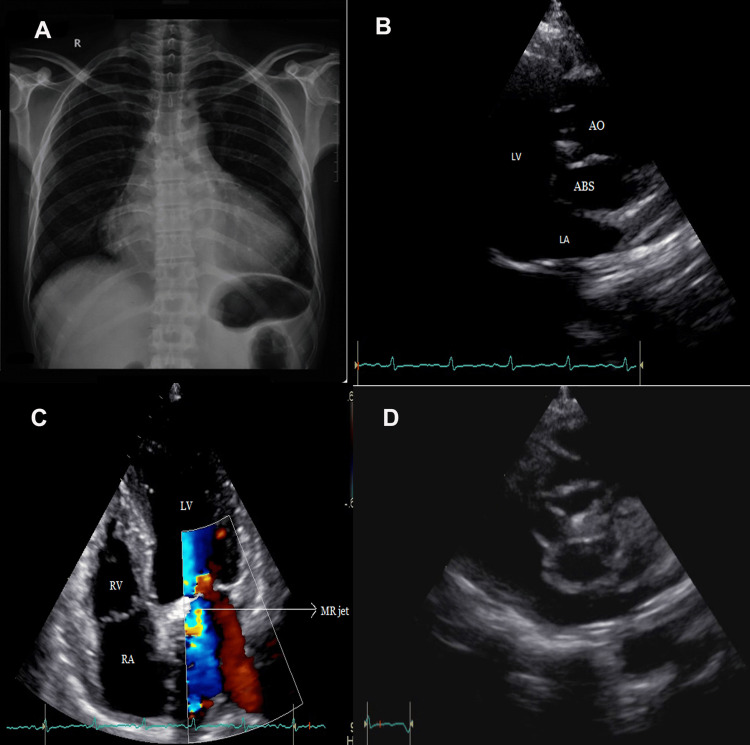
Chest radiograph showing cardiomegaly The TTE parasternal long-axis view shows an echo-lucent space (denoted by 'ABS') adjoining the area of MAIVF (A), and this space lies posterior to the aorta, bulging into the left atrium (B). The apical four-chamber view shows the jet of mitral regurgitation (C). The parasternal short-axis view reveals the bicuspid aortic valve (D). MAIVF: Mitral-aortic intervalvular fibrosa, TTE: Transthoracic echocardiogram, LV: Left ventricle, RV: Right ventricle, RA: Right atrium, LA: Left atiruma, MR: Mitral regurgitation
